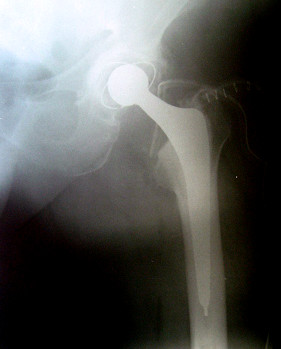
X-ray examination of total hip replacement patient during surgery after loss of femoral head.
